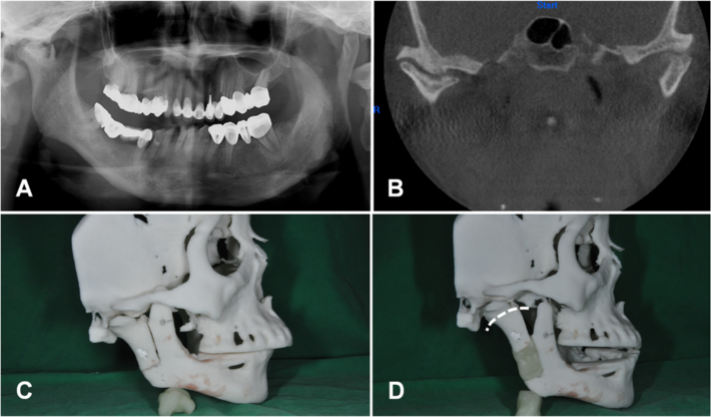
a The panoramic radiograph showed right TMJ ankylosis and facial asymmetry. b Coronal CT image shows fibrous adhesion around the right TMJ. c Reverse L-shape osteotomy line for distraction osteogenesis. d Distractor applied on rapid prototype model, and proposed line of osteotomy (*white dotted line*) for ankylosis release. A 23-mm distraction of the condyle-ramus segment was planned
